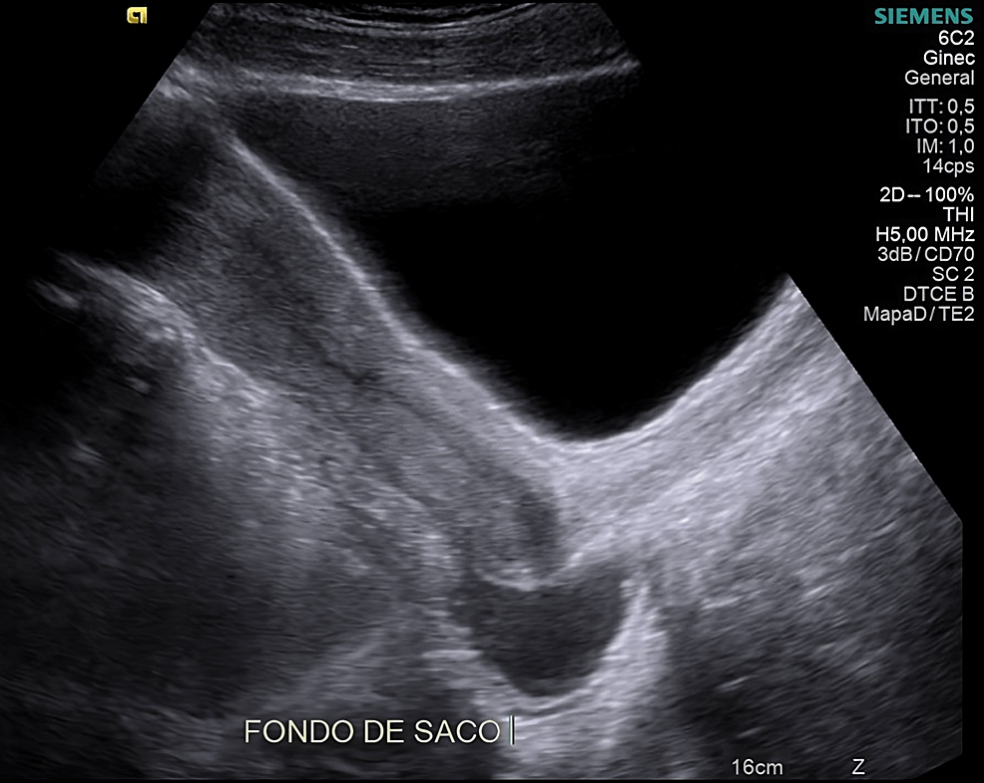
Pelvic ultrasound showing laminar fluid in Douglas’ pouch
